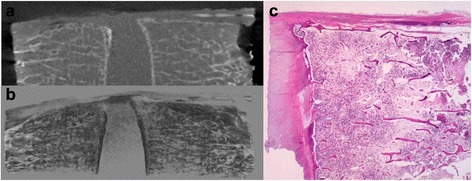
a–c Representative case of the posterior-vertebral type. a CT image: The ligament remained nearly unchanged with regard to thickness and surface area. Localized high-density areas were observed along the longitudinal axis in the PLL. b Three dimensional reconstruction image: High-density areas were without continuity with the vertebra. c Pathological image: This type showed isolated calcifying foci characterized by a linear-shaped, stereoscopically plate-shaped calcification and/or ossification in the PLL developed just behind the vertebral body
